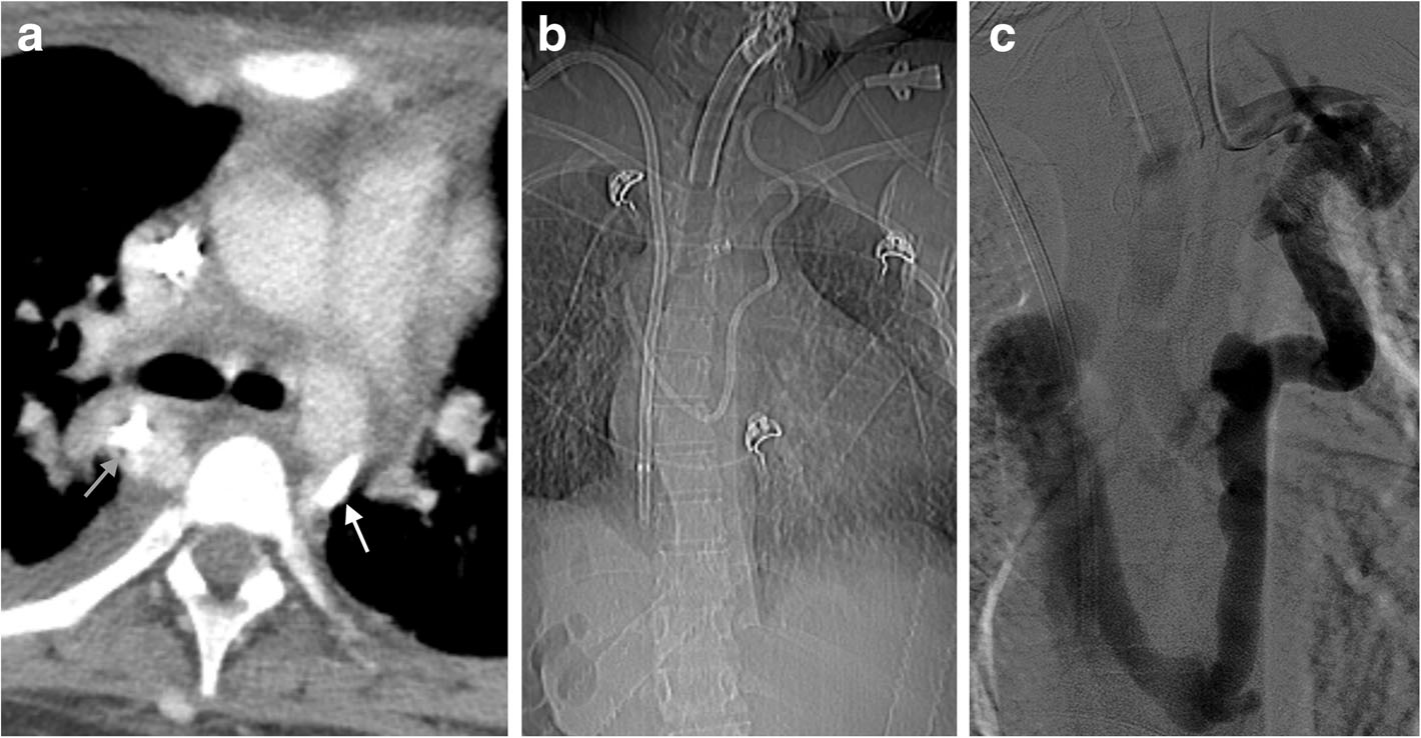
Collateral placement of central lines. a Axial CT image demonstrating placement of a central line through the hemiazygous (white arrow) and azygos vein (gray arrow). b CT scout image showing the tortuous path the line takes through the collateral vessels to the right atrium. c Interventional venogram of the same patient demonstrating collateral placement of the line
